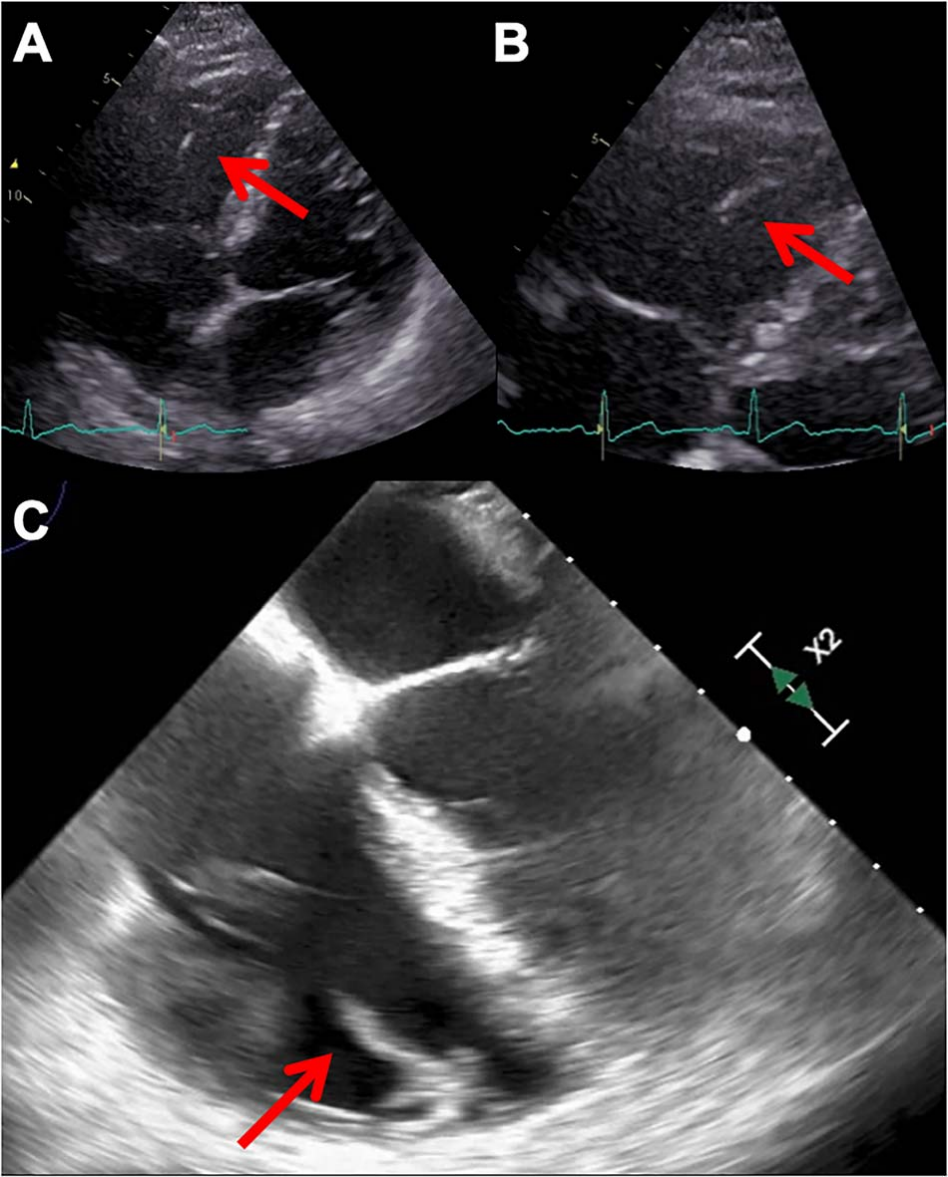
Echocardiographic visualization of suspected vegetation. Panels (A) and (B) display TTE images, with arrowheads denoting the suspected vegetation located in the apex of the right ventricle. Panels (C) provides TEE images, where arrowheads indicate the presence of a rod-like structure, raising the suspicion of vegetation.
